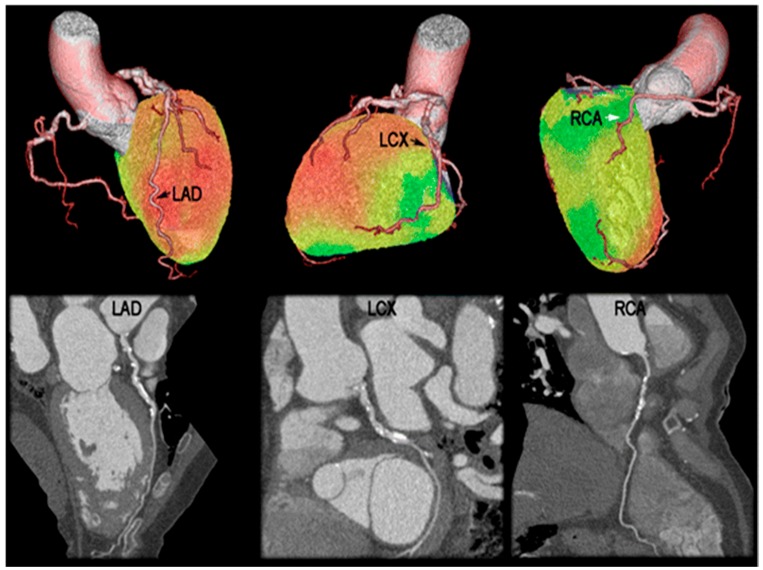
PET/CT assessing perfusion and coronary artery anatomy. Hyperemic MBF by PET (top) was reduced in the territories supplied by the left circumflex artery (LCX) and right coronary artery (RCA) (1.8–1.9 mL/min/g), but normal in the left anterior descending (LAD) arterial territory (2.6 mL/min/g). CCTA interpretation (bottom), however, suggested significant stenoses in the LAD, LCX and RCA. Adapted from Thomassen et al., 2013, by permission of *Eur. J. Nucl. Med. Mol. Imaging* [96].
